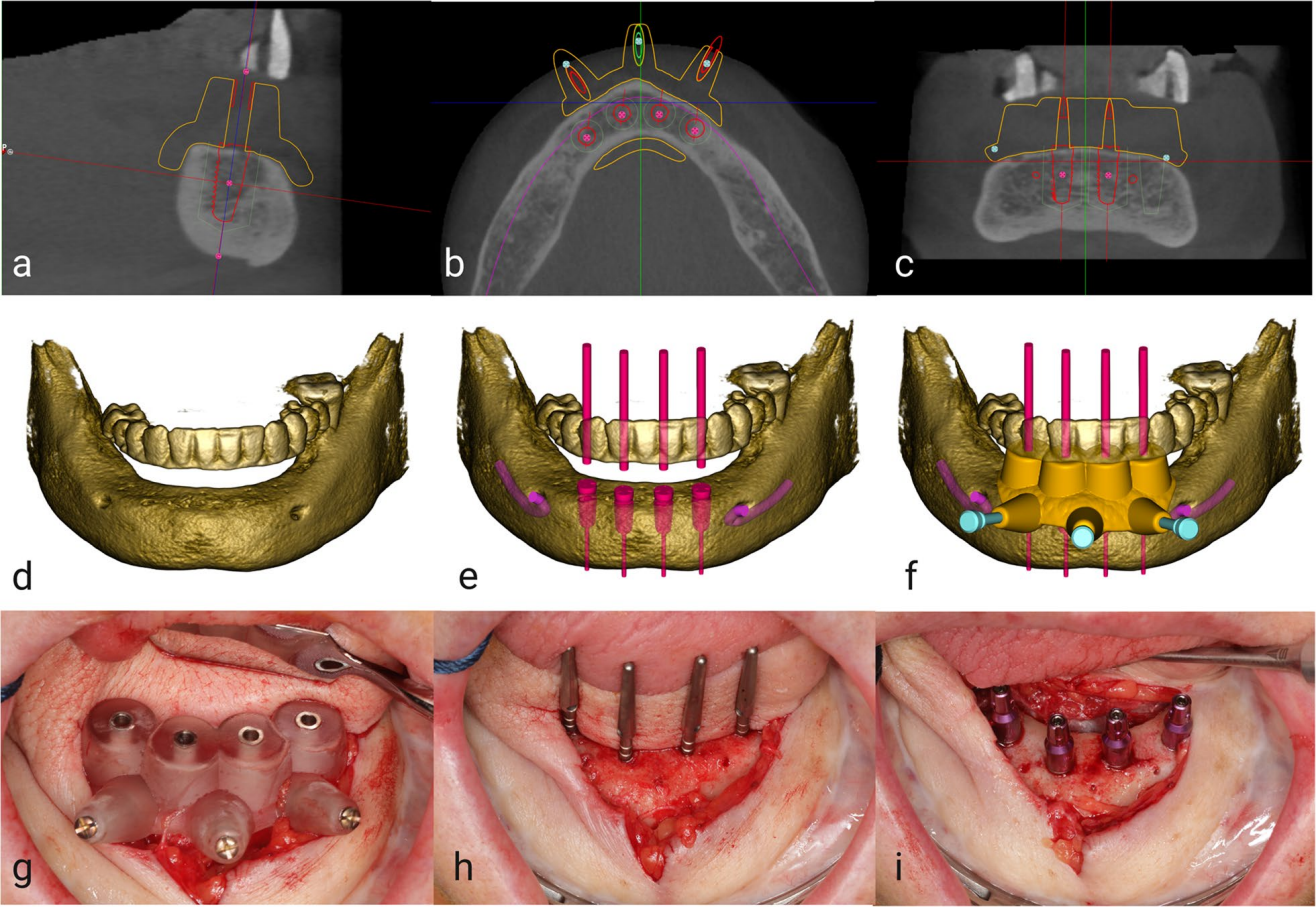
Case series of a bone-supported surgical guide, designed and fabricated using CBCT. Outline of the surgical guide and CBCT in sagittal (a), axial (b), and coronal (c) plane. Mandibular segmentation with prosthetic setup (d), implant position (e), and 3D surgical guide (f). Clinical images of implant placement with the surgical guide in situ (g), drilled implant positions with direction markers (h), and implants in situ (i)
